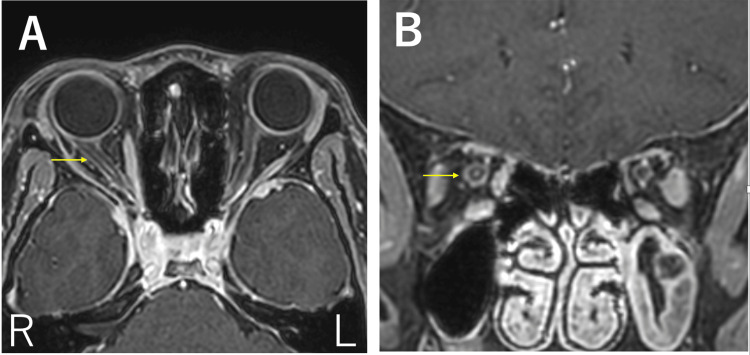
Contrast-enhanced brain MRI on hospital day 7 T1-weighted images show right optic nerve enlargement with internal contrast enhancement (arrows, A: axial; B: coronal, 1.5T). MRI, magnetic resonance imaging
